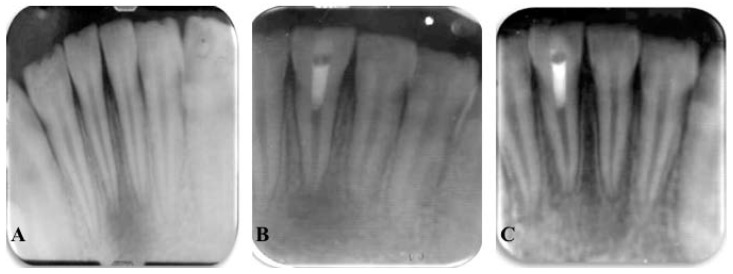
Radiographs of revascularized human mature teeth #25. (A) Preoperative radiograph to show inflammatory periapical lesion; (B) Postoperative radiograph after regenerative endodontic procedures; (C) At 12-month follow-up, resolution of apical periodontitis [61].
